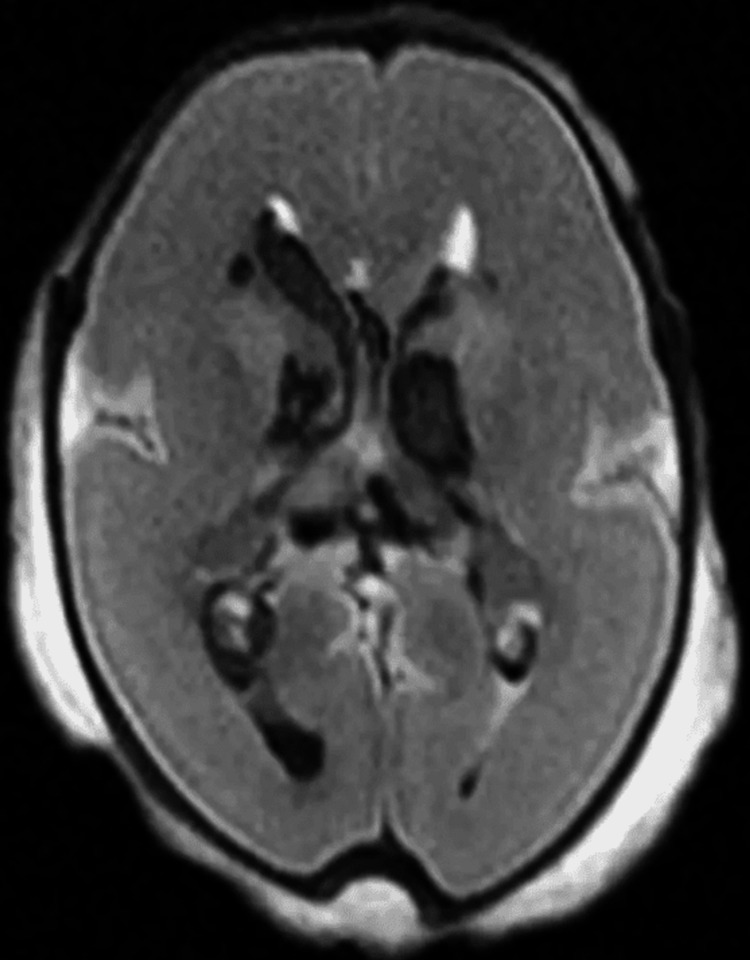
Axial T2-weighted postmortem MRI of brain in a 23-week fetus demonstrates bilateral intraventricular and periventricular hemorrhage.
